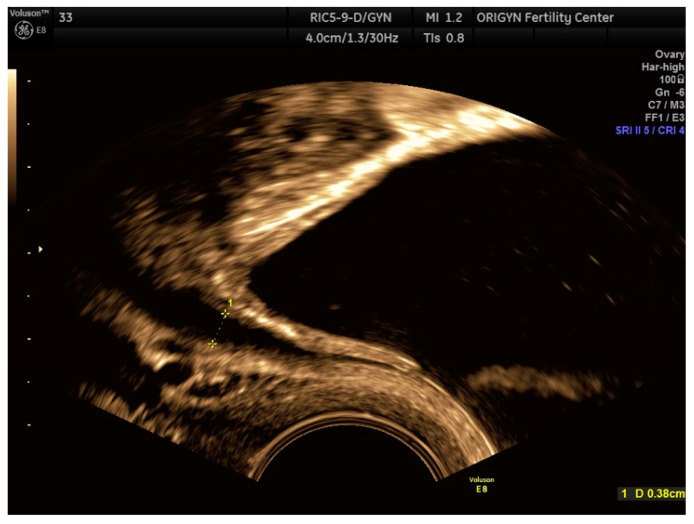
Transvaginal ultrasound of a normal ureter during peristalsis. Notice the flow of urine into the bladder.
